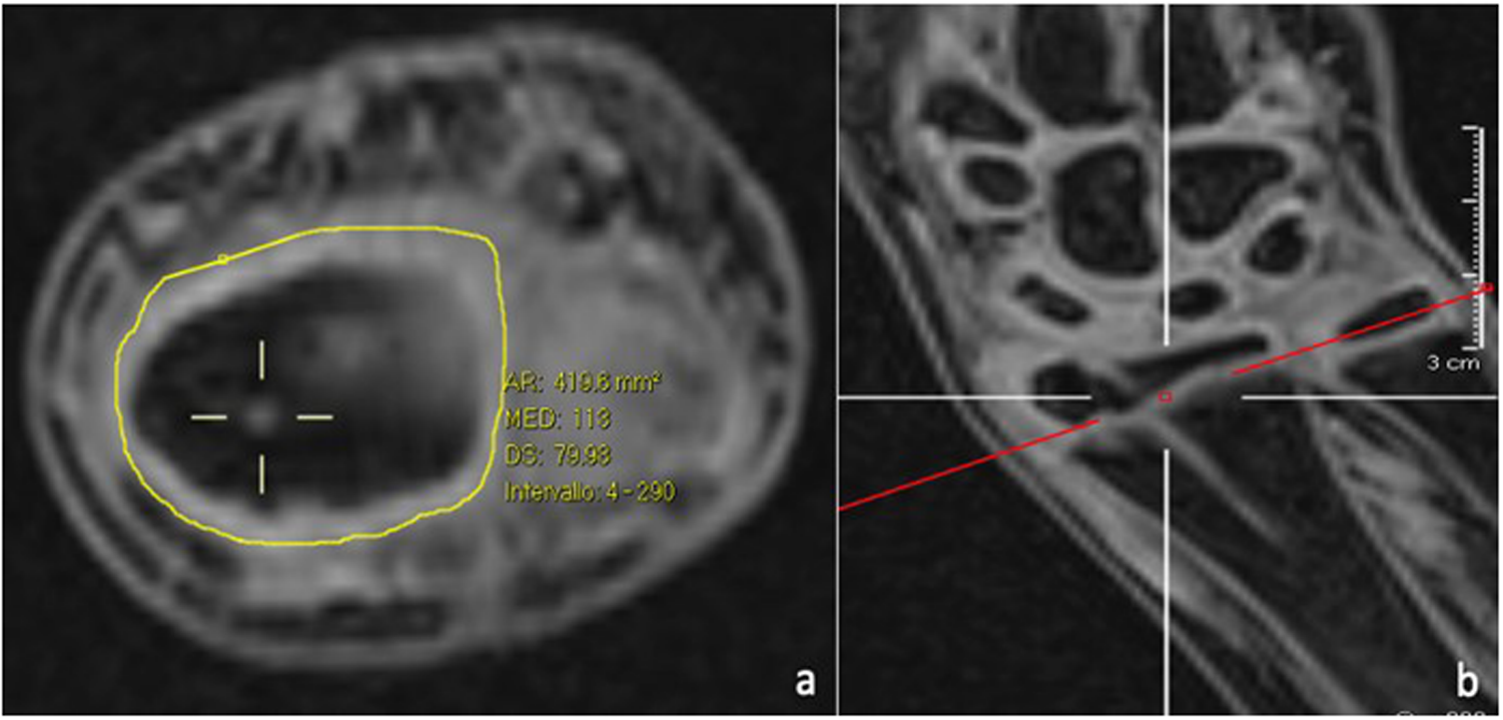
The transphyseal lesion after K-wire removal, as seen on MRI axial (a) and coronal (b) views, is estimated as a percentage of the total surface are of the physis.
